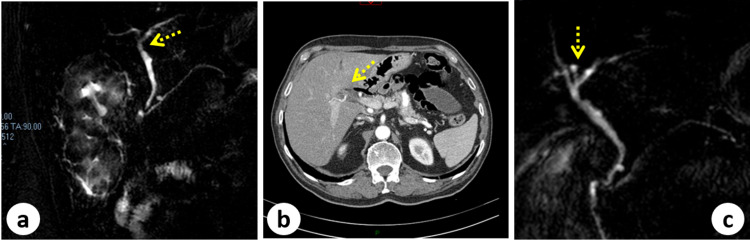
Radiological images: (a) MRCP showing GA (dotted arrow); (b) CT scan image showing right hepatic artery and its relation with right hepatic biliary duct (dotted arrow); (c) MRCP showing GA and the biliary variant of the posterior right hepatic duct with its insertion into the left hepatic duct (dotted arrow). MRCP: magnetic resonance cholangiopancreatography, GA: gallbladder agenesis
